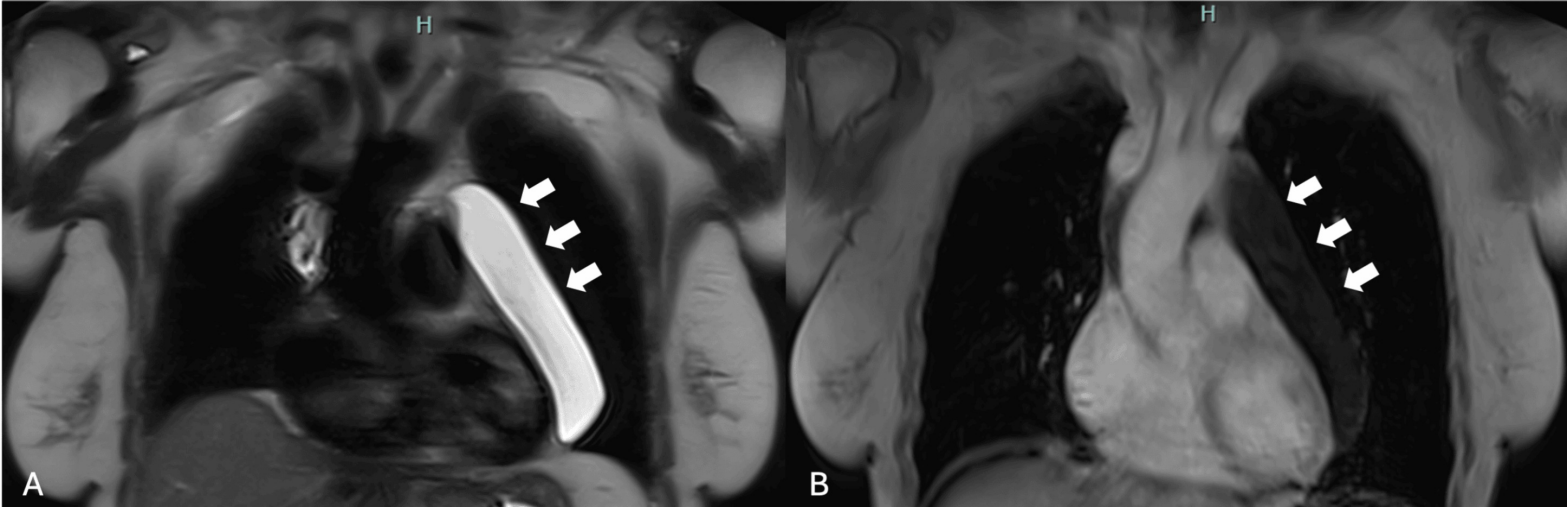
(A) Coronal T2-weighted MR image shows a well-defined lesion (white arrows), with thin walls and homogenous high signal intensity. (B) Coronal T1-weighted MR image shows the lesion with low signal intensity (white arrows). MR: magnetic resonance
